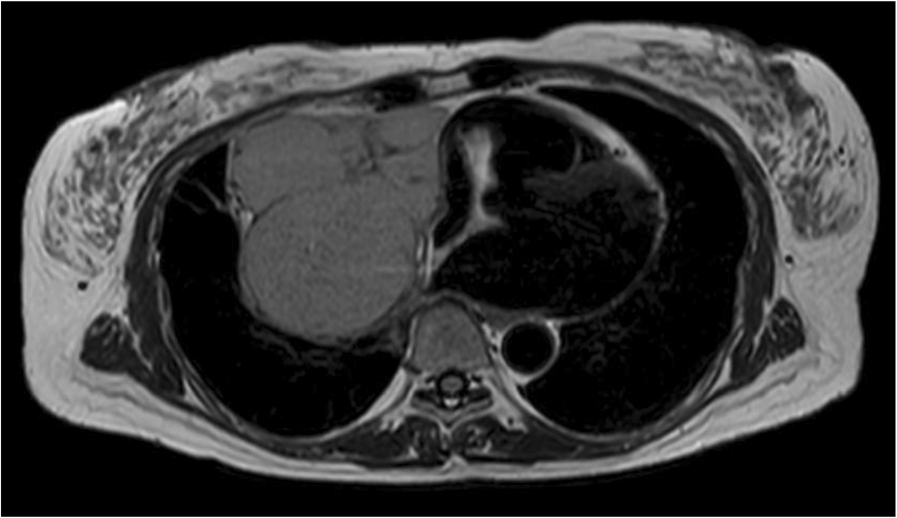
Magnetic resonance imaging (T2-weighted imaging) shows a capsulated mass (10 × 11 cm in diameter) at the right anterior mediastinum
